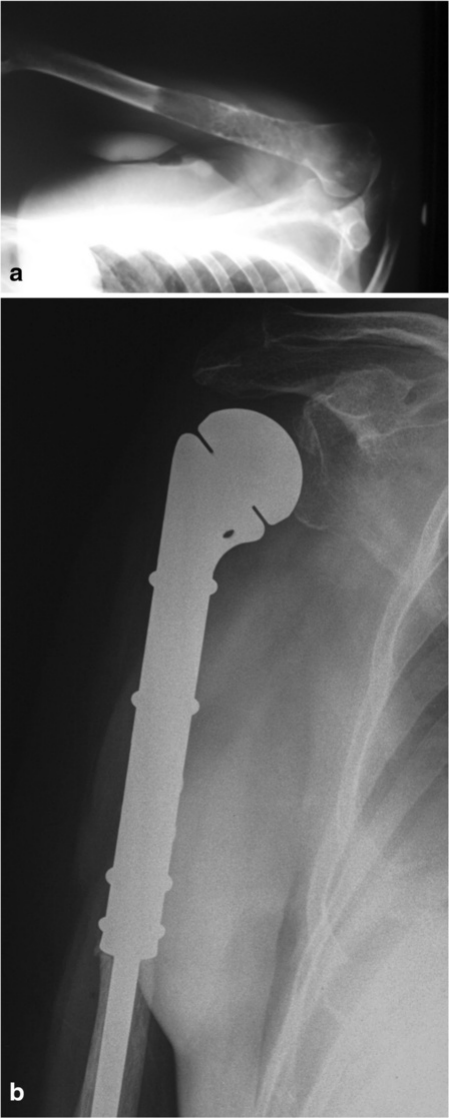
Radiographs of proximal humerus destruction by myeloma (a) and modular endoprosthetic replacement (b)
